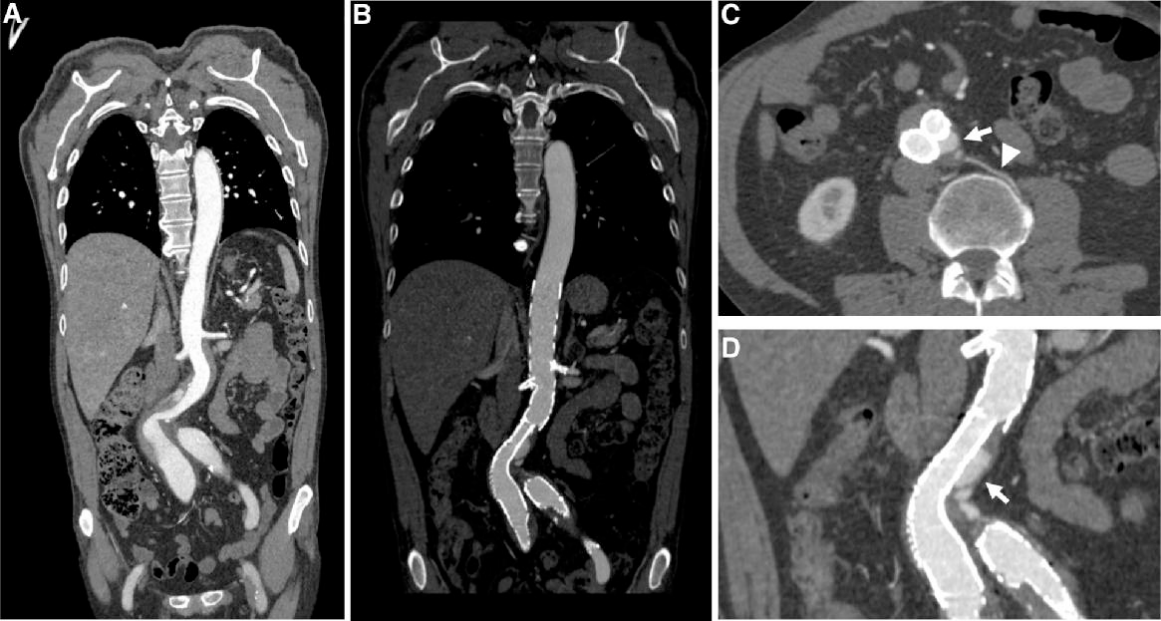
Computed tomography angiography studies of abdominal aorta disease. (*A*) Infrarenal abdominal aorta dissection extending to the right common iliac artery and bilateral common iliac artery aneurysms. (*B*) Endovascular abdominal aortic repair with branched and bifurcated custom-made endograft with Zone 4 Ishimaru’s proximal landing. (*C* and *D*) Type II distal graft endoleak (white arrows) due to backfilling of the aneurysm sac through a lumbar vessel (head arrow).
